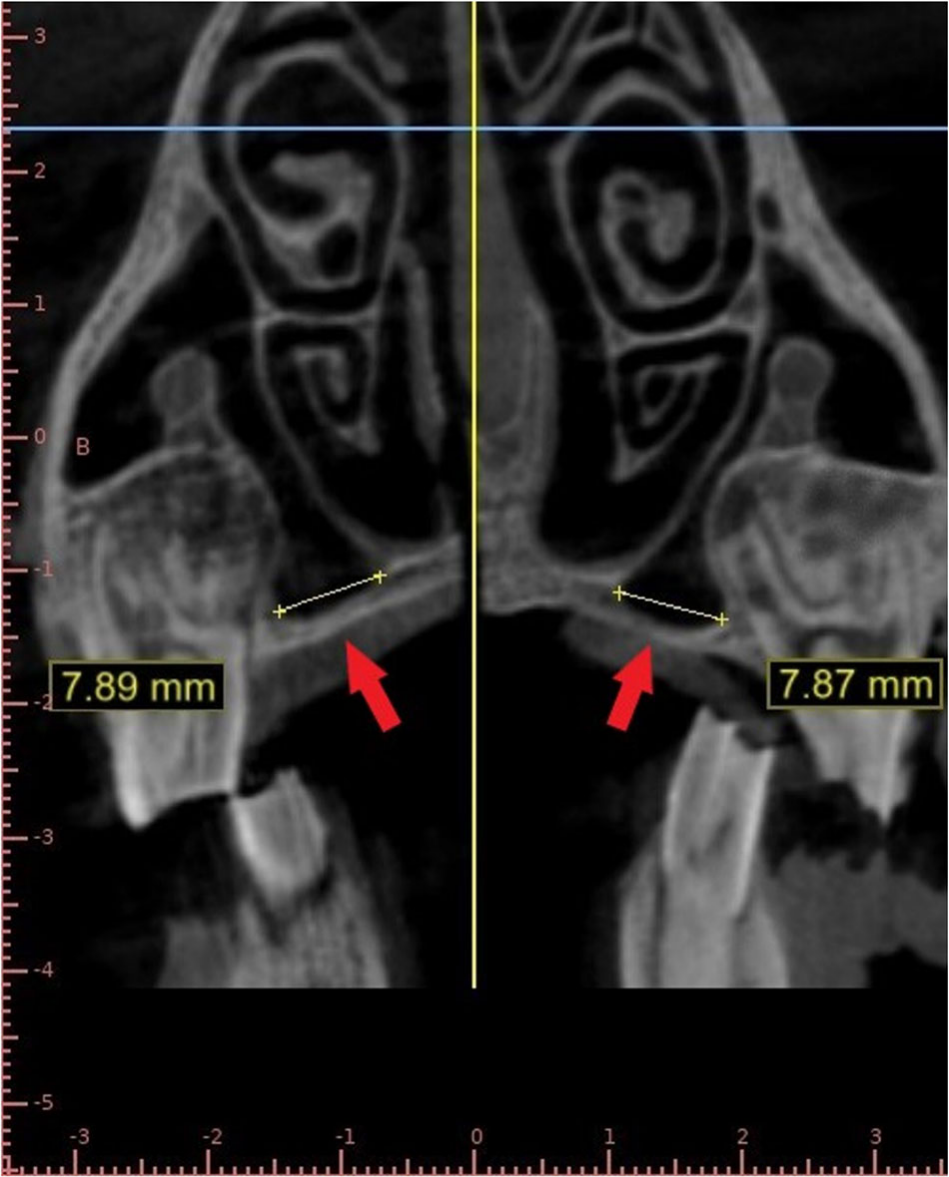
Entry point on CBCT image. Entry point shown on CBCT scan marked by the red arrows.
